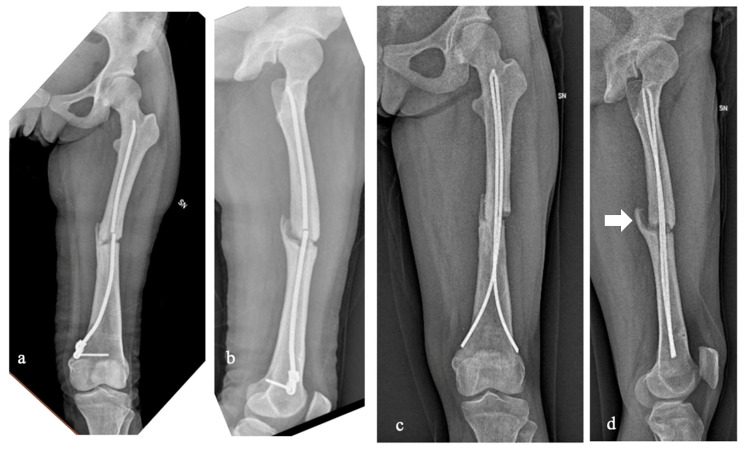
Case 5 had a displaced diaphyseal left femur fracture that never healed and, as a result of a low-energy traumatic event, the femur refractured at the pseudoarthrosis site with associated nail breakage (a,b). We decided to treat the refracture with two 4 mm titanium elastic nails placed with the standard technique (ESIN, elastic stable intramedullary nailing). Within a year from the surgery, the X-rays showed a hypertrophic pseudoarthrosis (arrow) that will require a new surgery (c,d).
